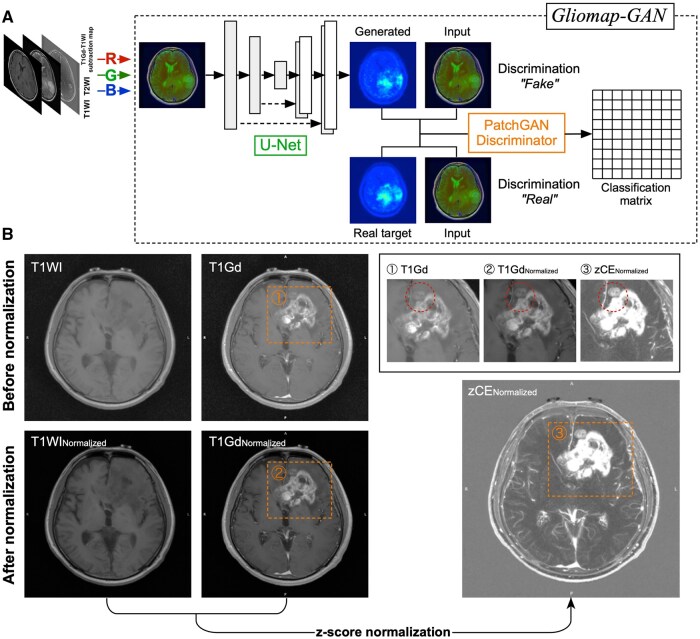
The pix2pix cGAN named *“Gliomap-GAN”* was trained using an RGB color-coded MRI derived from a normalized T1WI, T2WI, and zCE. The target image was the corresponding normalized MET-PET. Further details of the network can be found elsewhere.^27^^,^^28^ (A) The entire procedure for creating zCE_Normalized_ from T1WI and T1Gd is presented. Both images were first normalized through the process described in Figure 1, followed by a z-score-based subtraction. The dynamic range of the subtraction image was further expanded from 0 to 255. A subtle contrast enhancement that is difficult to identify in the original image (1) can be identified in zCE_Normalized_ (3), highlighted with a red dotted circle. (B).
